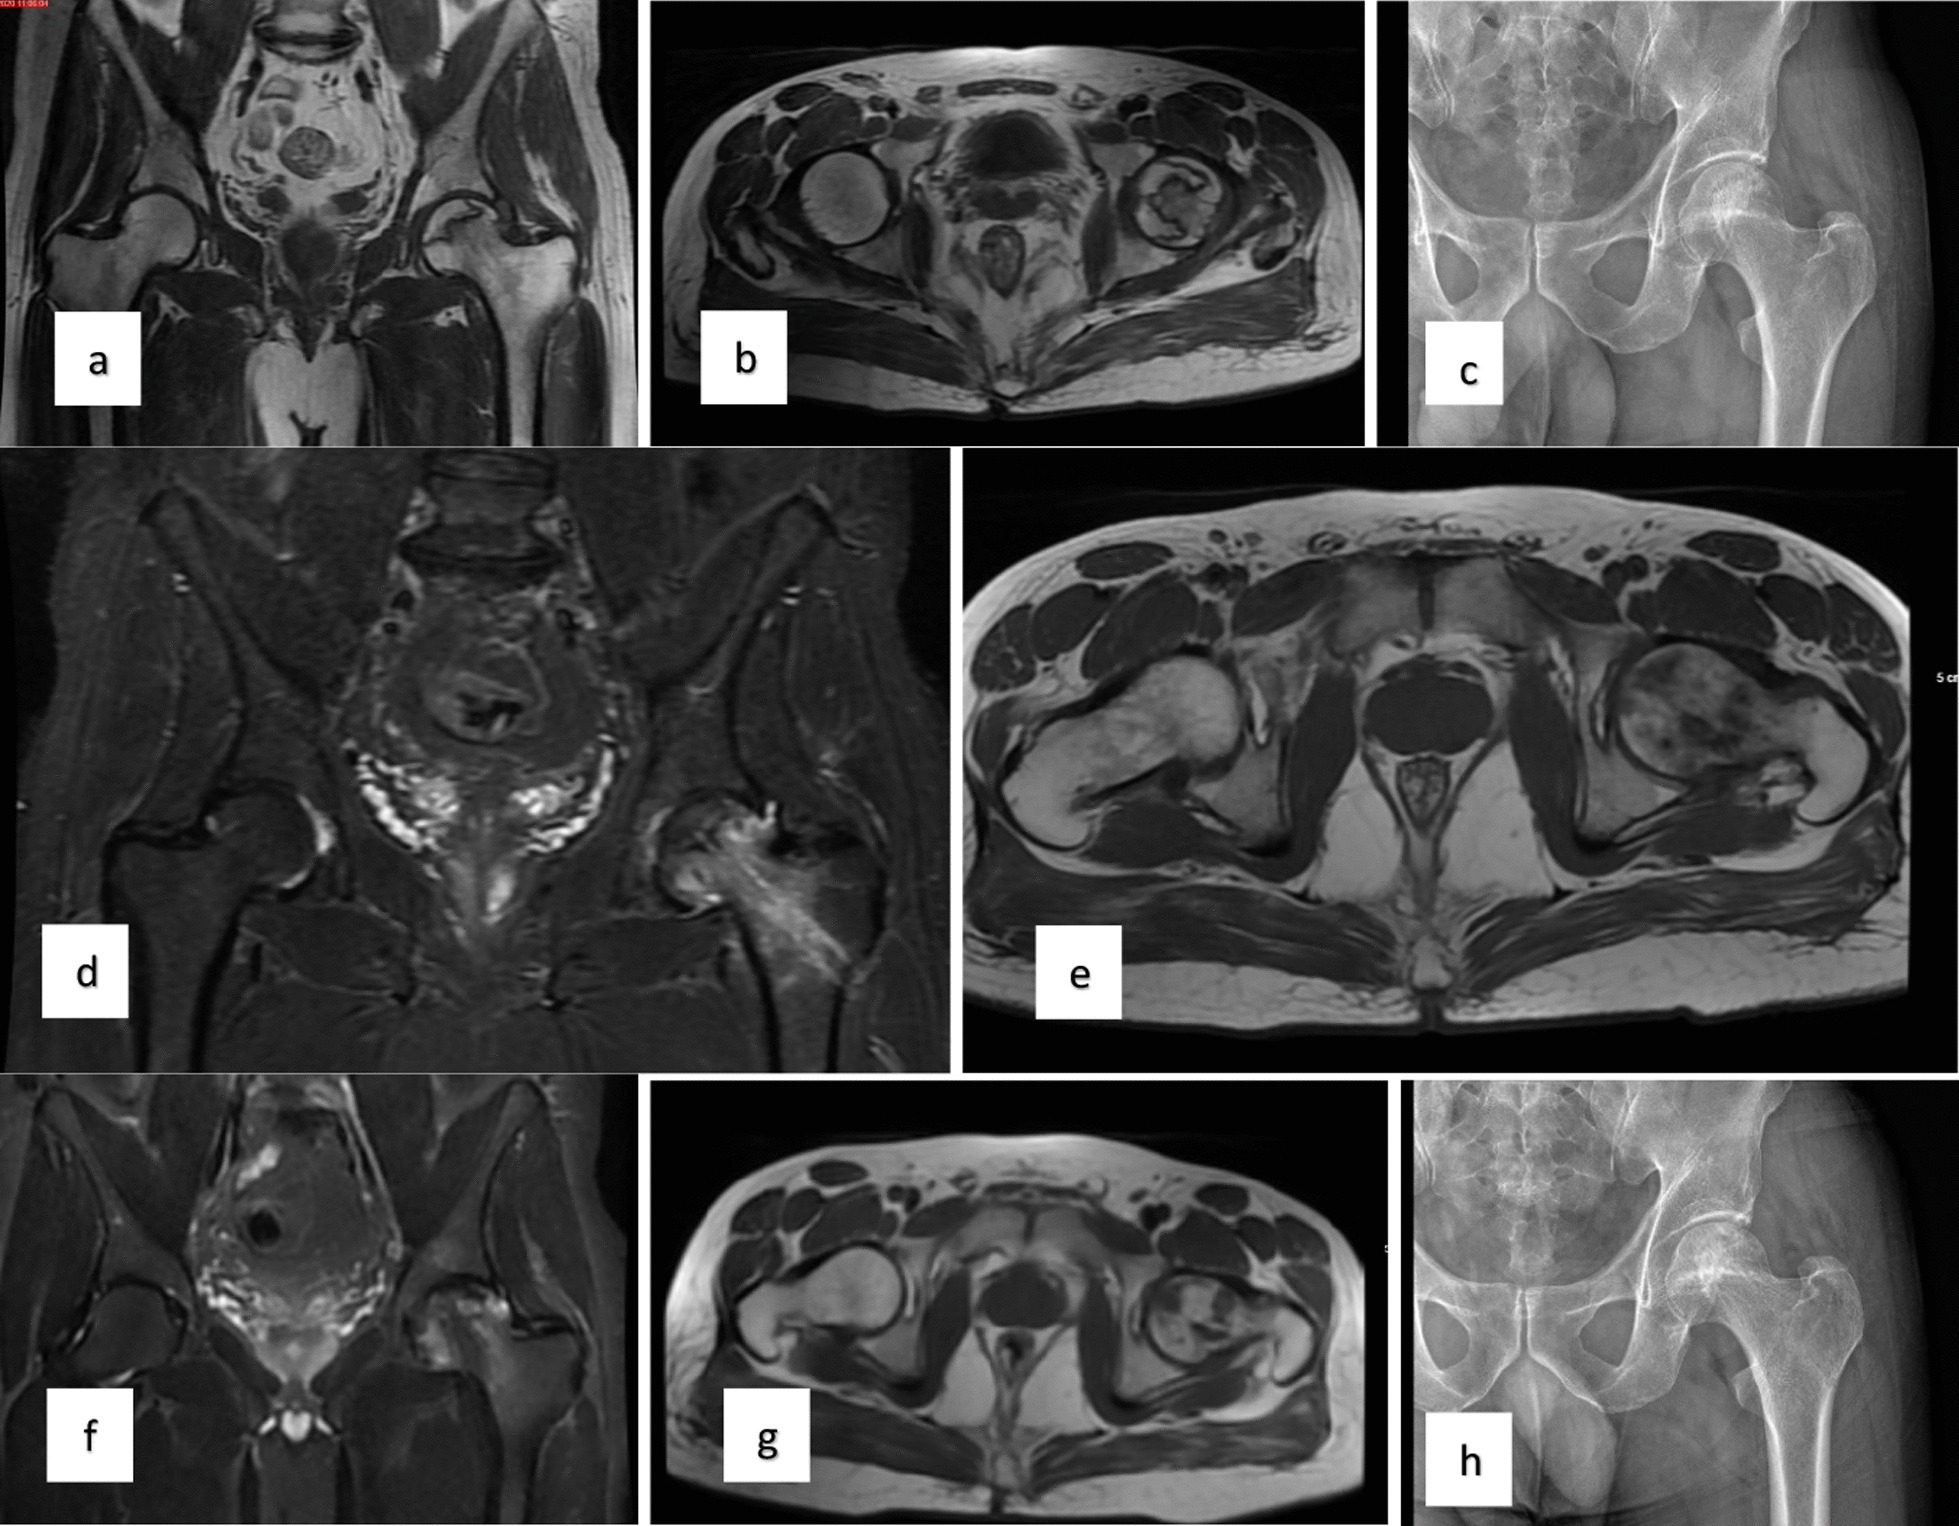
a–c Preoperative MR and X-ray images of the patient with left femoral head avascular necrosis. d, e MR images at 3 months after surgery f–h: MR and X-ray images at second year after surgery
